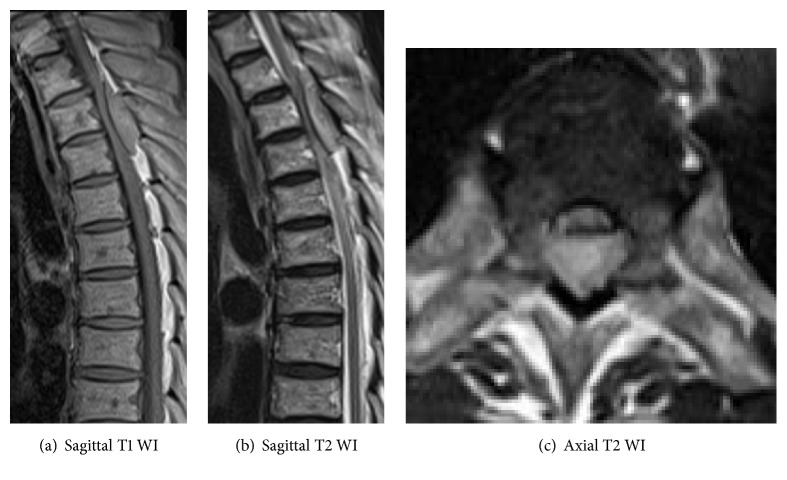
Thoracic spine MRI before operation. A thoracic epidural mass lesion was evident showing low intensity on the T1 weighted image and iso-high intensity on the T2 weighted image.
